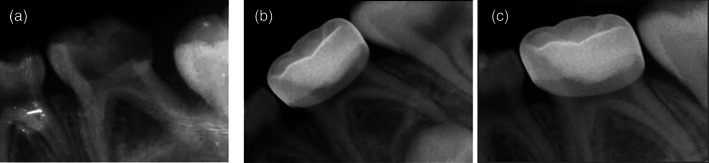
Successful TAP pulp therapy in a lower second molar with deep carious lesion and bifunctional radiolucency. (a) Pre‐operative baseline radiograph. (b) Six‐months follow‐up radiograph showing reduction in the size of the bifurcation radiolucency. (c) Twelve‐months follow‐up showing complete healing of the radiographic radiolucency
